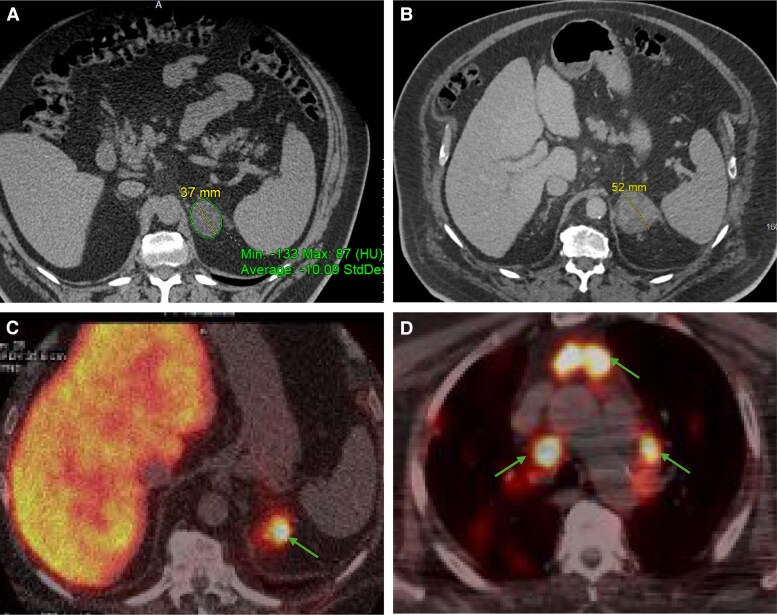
Imaging studies for case 1 showing characterization as a lipid-rich adenoma (A), followed by diagnosis of ACC (B-D). (A) Noncontrast CT performed at year 4 showing a 37-mm left adrenal nodule with density −10 HU. (B) Contrast-enhanced CT at year 14 showing an increase in lesion size to 52 mm. (C) [^11^C]Metomidate PET-CT showing an avidly enhancing left adrenal lesion (green arrow) with (D) multiple [^11^C]Metomidate avid thoracic nodal metastases (green arrows).
